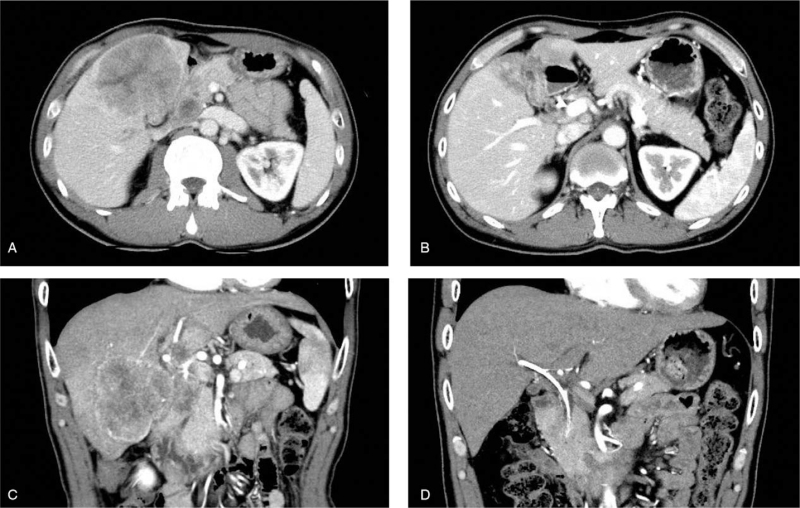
Contrast-enhanced computed tomography (CT) findings pre- and post-chemotherapy. (A, B) Axial portal venous phase. (C, D) Coronal arterial phase. (A, C) Pre-chemotherapy. (B, D) After 5 cycles of chemotherapy. (D) The plastic stent placed in the stenotic common bile duct is visible. Five courses of chemotherapy markedly reduced both liver tumor and lymph node sizes.
